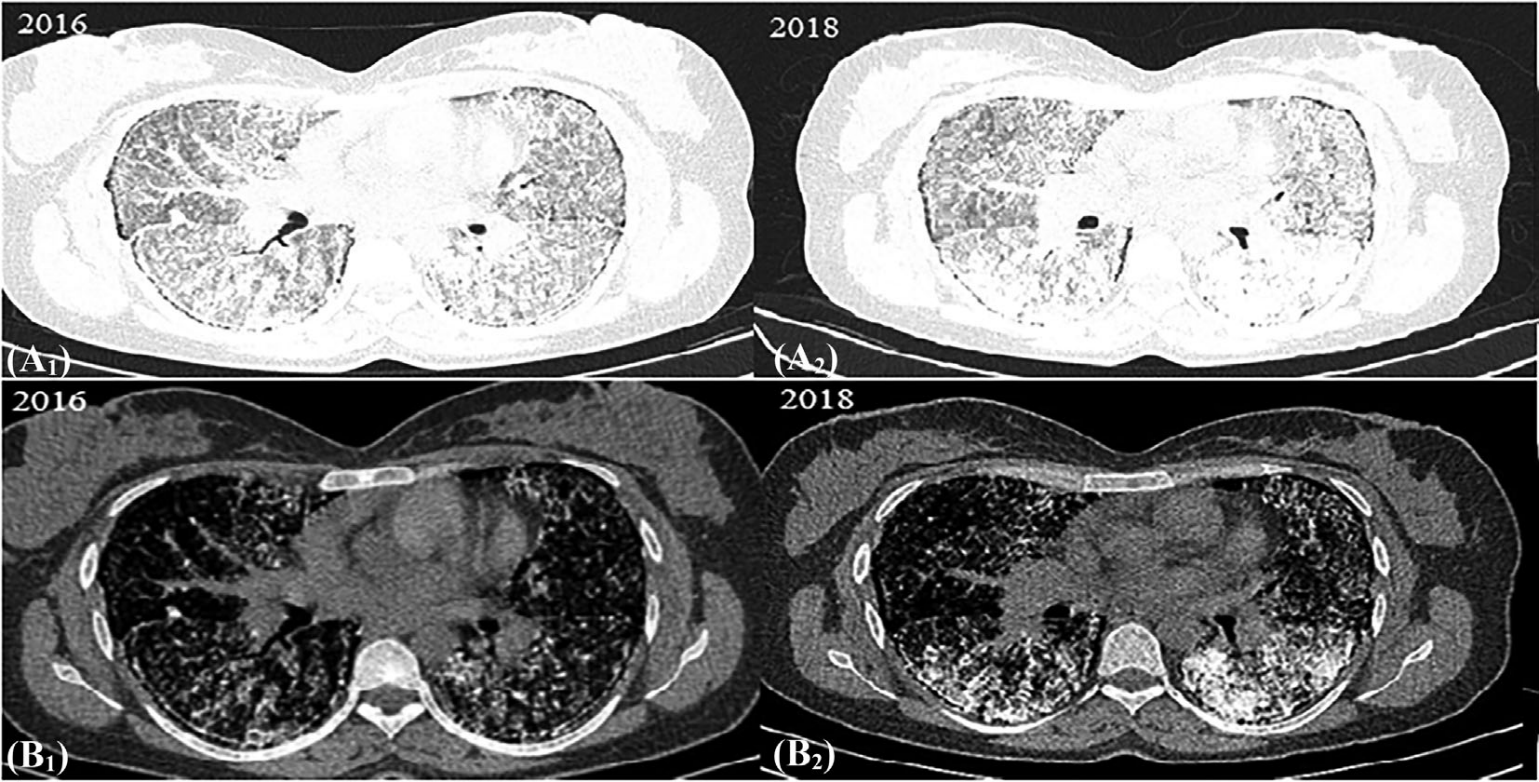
High‐resolution computed tomography of the patient with pulmonary alveolar microlithiasis over 2 years (A_1_, A_2_) a section of the lung in a pulmonary window setting, demonstrating expansion of fibrosis over the years (B_1_, B_2_) a mediastinal section of the lung, showing an increased number and size of calcified foci in B_2_.
